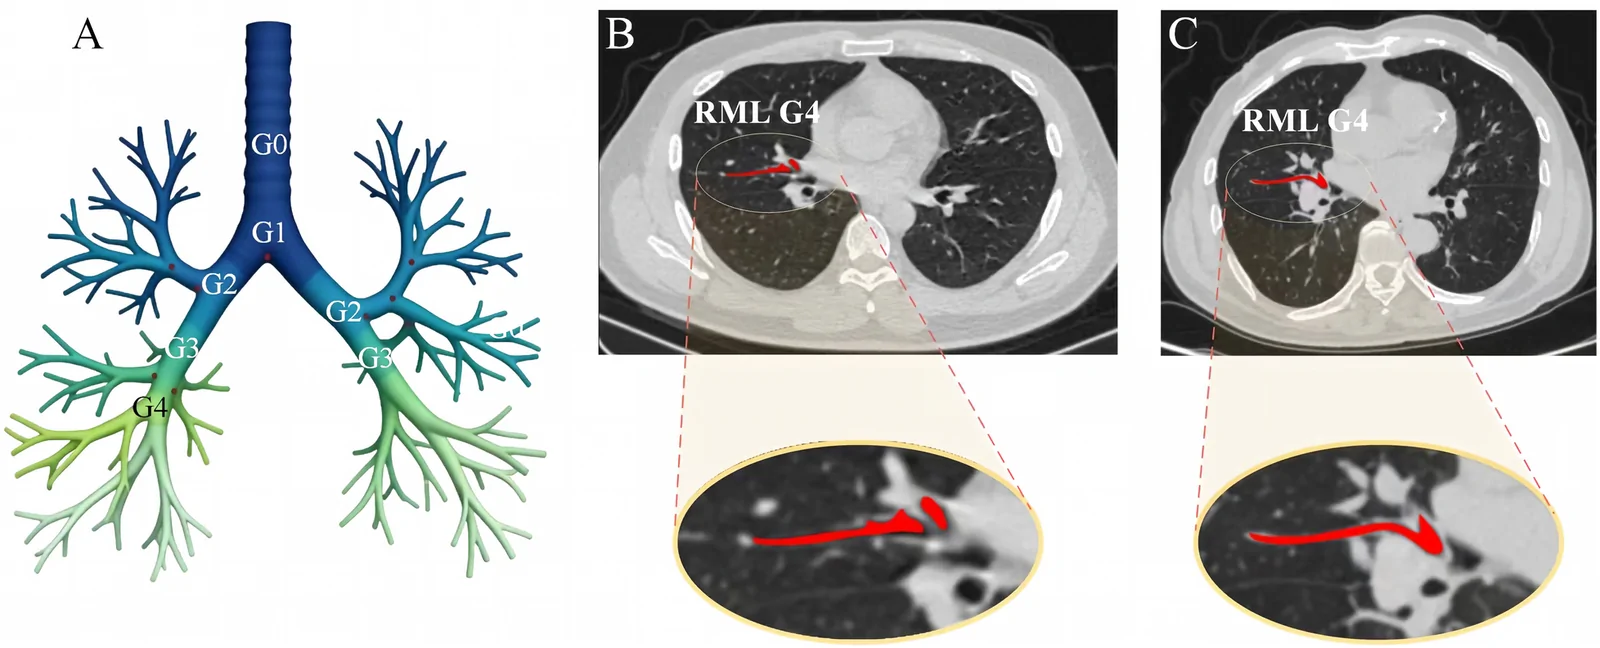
Representative chest CT images of a Non-COPD control and a patient with NE-COPD. (A) Axial chest CT image of a Non-COPD; (B) Axial chest CT image of a patient with NE-COPD; (C) The location of the fourth-generation airway in the right middle lobe (RML G4) is indicated in red in both panels.
